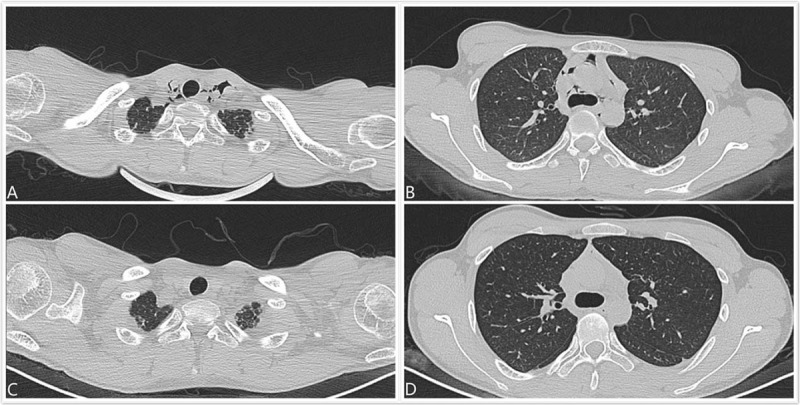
HRCT of the lung upon admission, showing gas in the (A) thoracocervical area and (B) mediastinum, and (C, D) significantly decreased gas in the same area after treatment at day 8 of admission.
